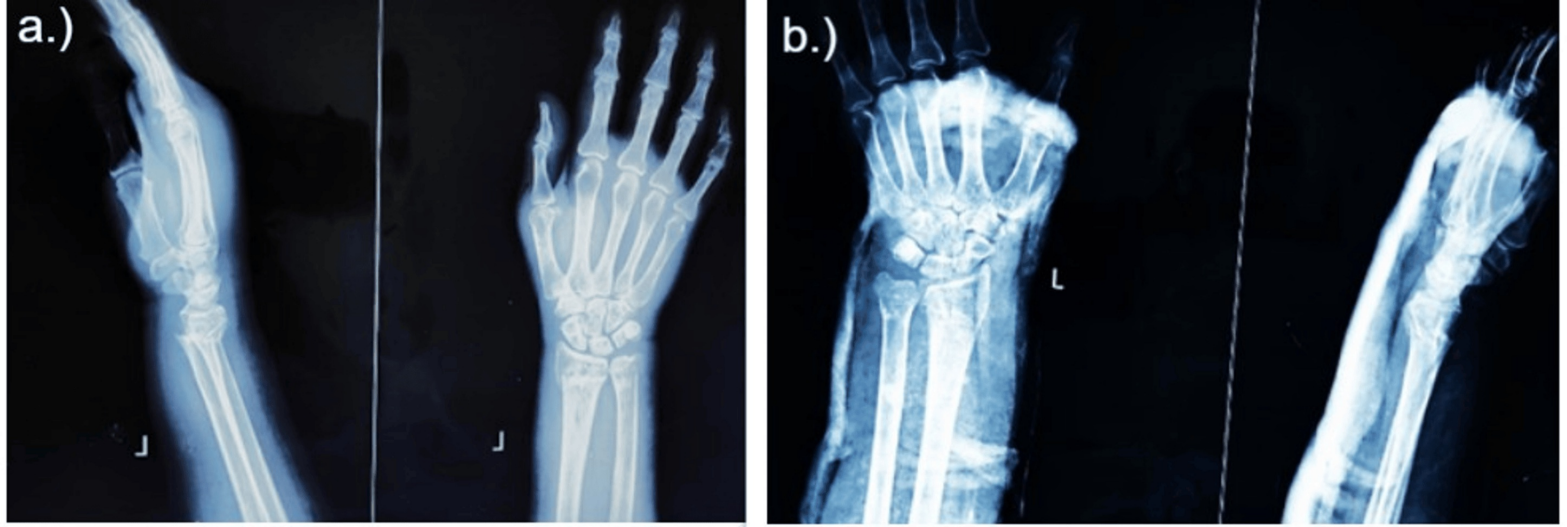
X-ray of the wrist: anteroposterior view and lateral view. A) Distal end radius fracture. B) After close reduction and Colle’s cast application.
